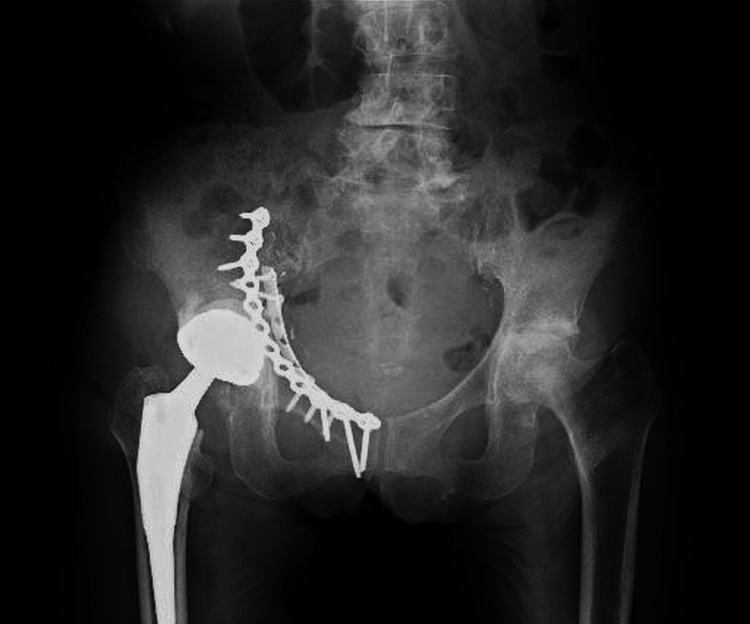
Postoperative plain radiograph of the pelvis (anteroposterior view). The fracture was stabilized using two locking plates placed anteriorly, and the acetabular component was revised with a cemented cup.
